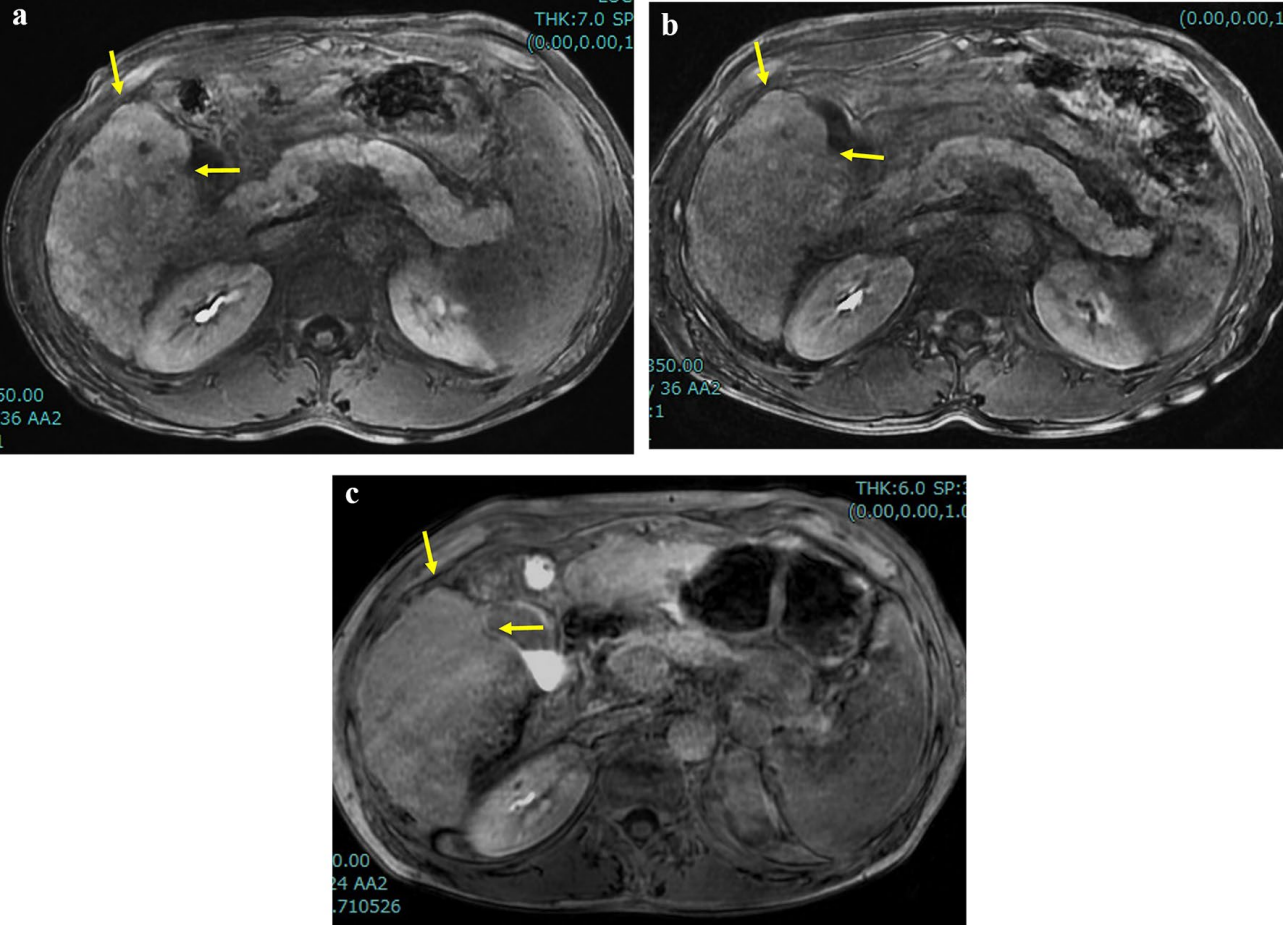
Longitudinal change of gallbladder fossa nodularity (GBFN) in 42-year-old man with alcoholic liver disease. a Hepatobiliary phase (HBP) of gadoxetate-enhanced MRI revealed grade 2 GBFN (arrows). Note slightly high signal intensity at the peritoneal side of GBFN. ALBI score and modified ALBI grade were − 1.7 and grade 2b. b HBP image one year later reveals less prominent GBFN, but grade 2 (arrows) was again assigned. Note the relatively high signal on the peritoneal side as observed in 5a is less prominent. ALBI score and modified ALBI grade were − 1.8 and grade 2b. c HBP image five years later (6 years from 5a) reveals grade 1 GBFN (arrows), exhibiting mostly iso-signal intensity as compared to the surrounding liver parenchyma. ALBI score and modified ALBI grade were − 1.4 and grade 2b, respectively, suggesting slight progression of liver dysfunction since 5a
